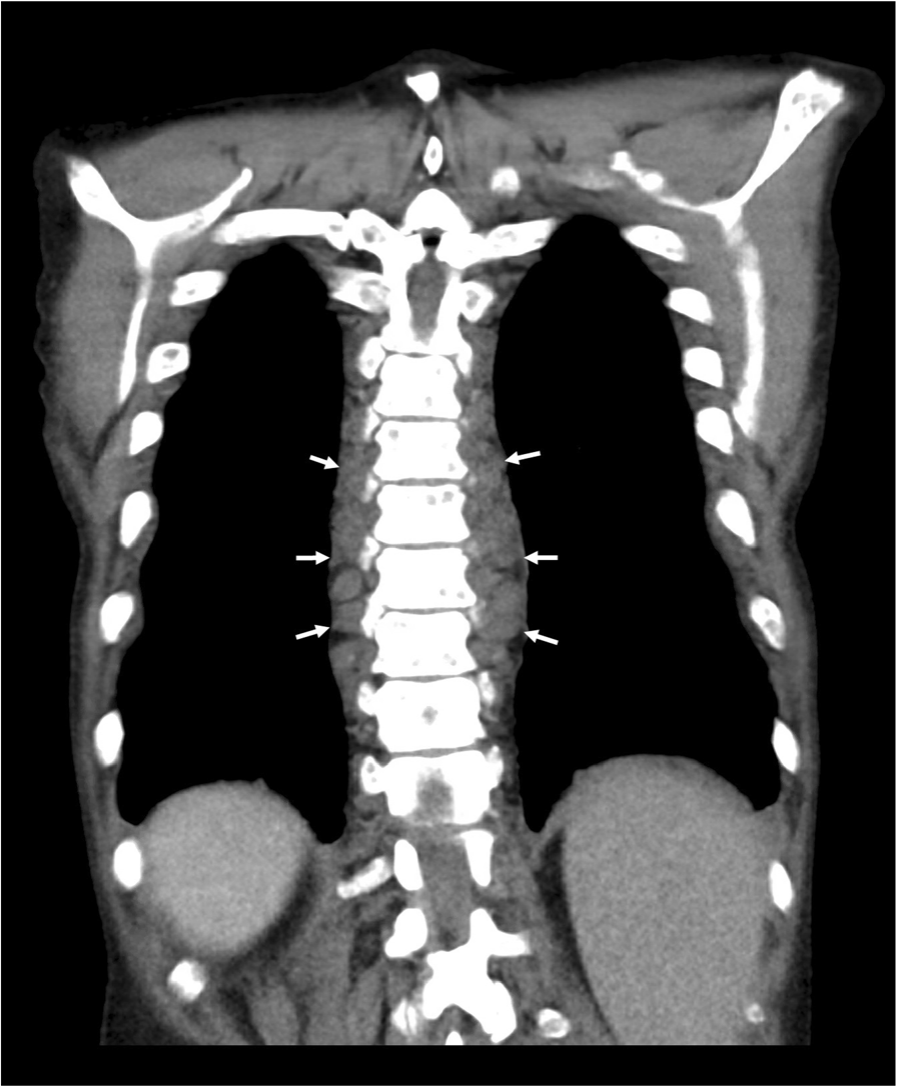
Coronal CT of the chest demonstrating extramedullary haematopoiesis in the posterior mediastinum, which typically appear as bilateral and symmetrical posterior mediastinal masses (white arrows)
